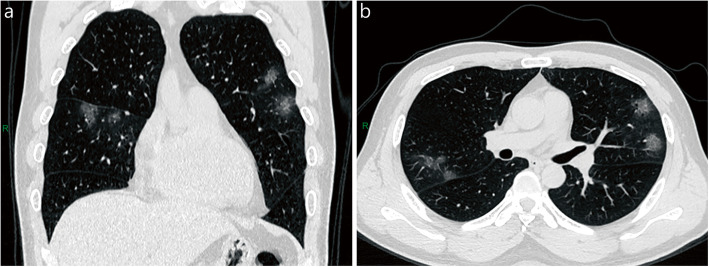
Chest CT of moderate patient. A 48-year-old male patient coughed for 1 week. Patchy ground-glass opacities were seen in the upper lobes of both lungs and the middle lobe of the right lung. Coronal (a) and axial (b) sections in lung window. R: right
